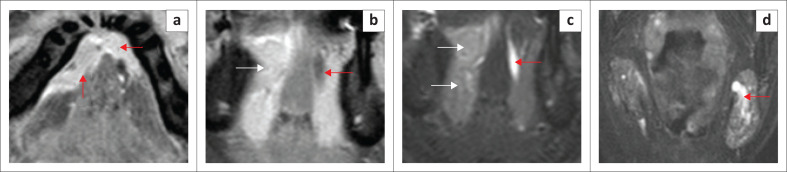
Stage T2 squamous cell carcinoma of the right floor of the mouth with enlargement of the right sublingual gland, crossing the midline and obstructing the left submandibular duct in a 68-year-old male. The T1 post-contrast fat-suppressed axial image (a) shows the tumour crossing the midline (red arrows). The T1 post-contrast fat-suppressed coronal image (b) demonstrates the less enhancing tumour component (white arrow) in contrast to the normal sublingual gland and the dilated left submandibular gland duct (red arrow). The T2-weighted short tau inversion recovery (T2W-STIR) coronal image (c) shows the tumour involving the right sublingual gland (white arrows) and a dilated distal left submandibular gland duct (red arrow). The T2-STIR coronal image (d) shows the enlarged left submandibular gland with a dilated ductal system (red arrow).
